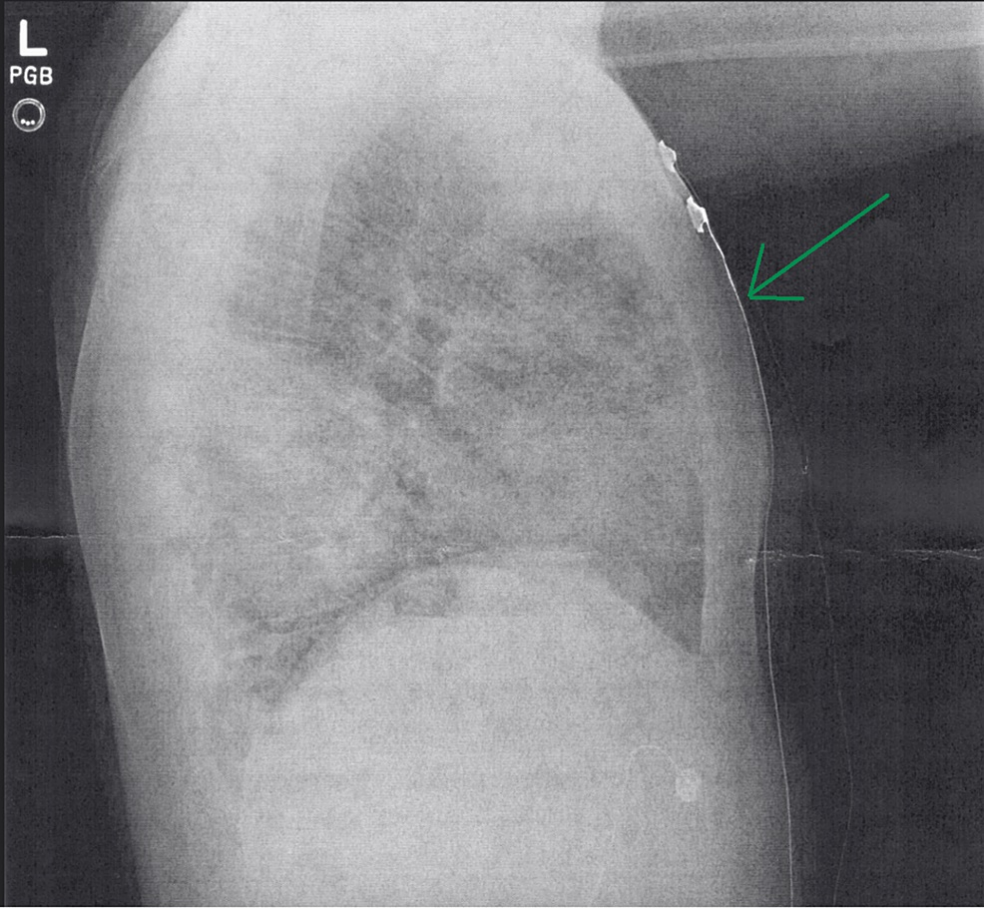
Lateral chest X-ray on repeat admission
